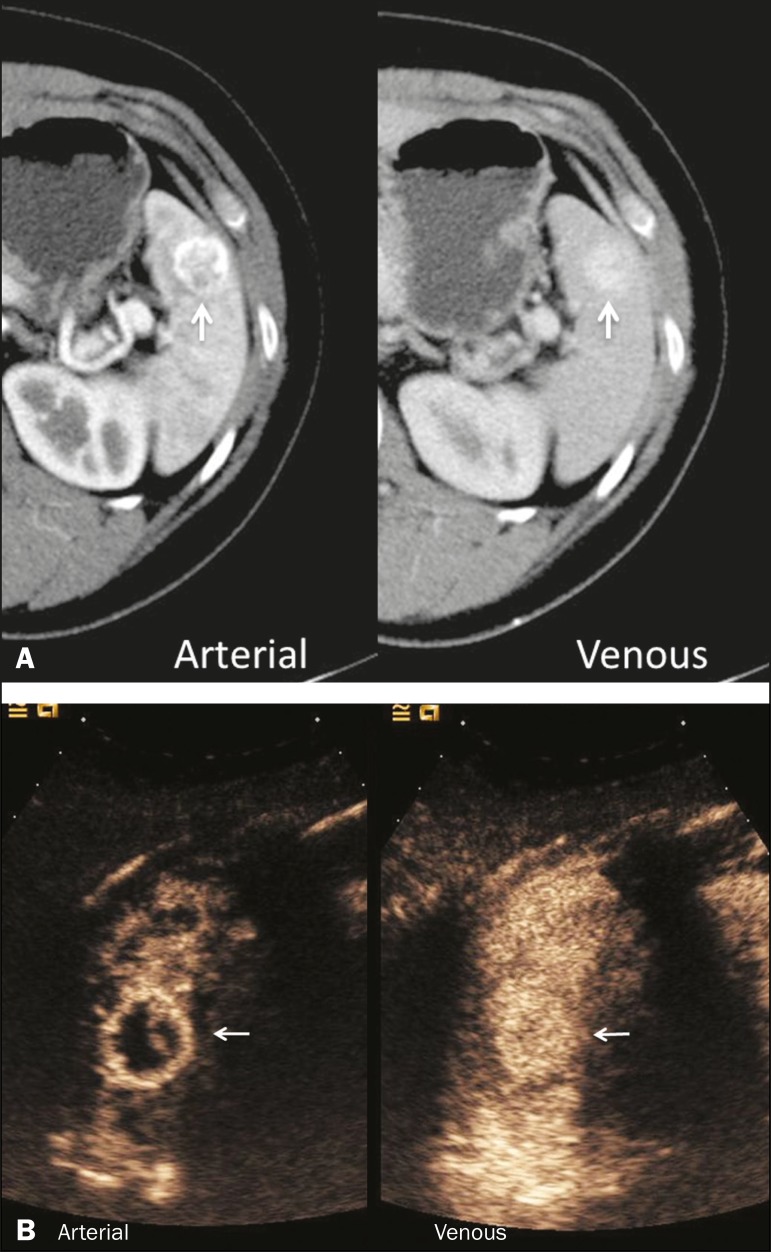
Splenic hemangioma. A: Contrast-enhanced CT scan showing
